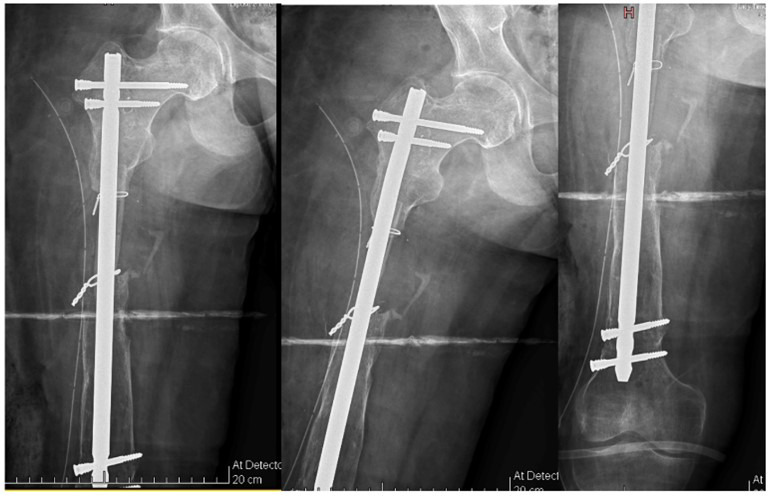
Postoperative radiograph of the right femur following definitive fixation with an intramedullary SIGN nail and reconstruction of the residual bone defect using a non-vascularized fibular strut graft.
